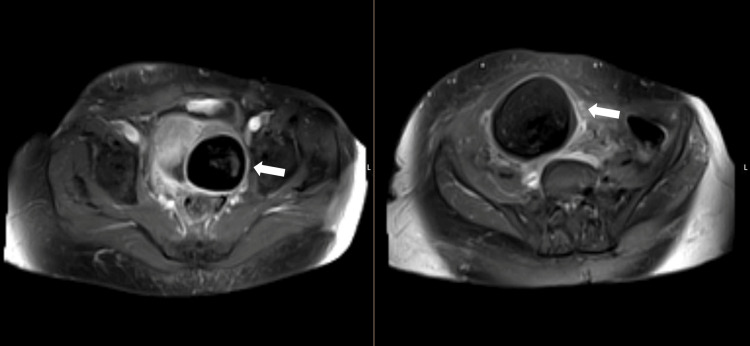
Axial MRI scan of the pelvis after gadolinium injection showing the enhancing component (arrows).
